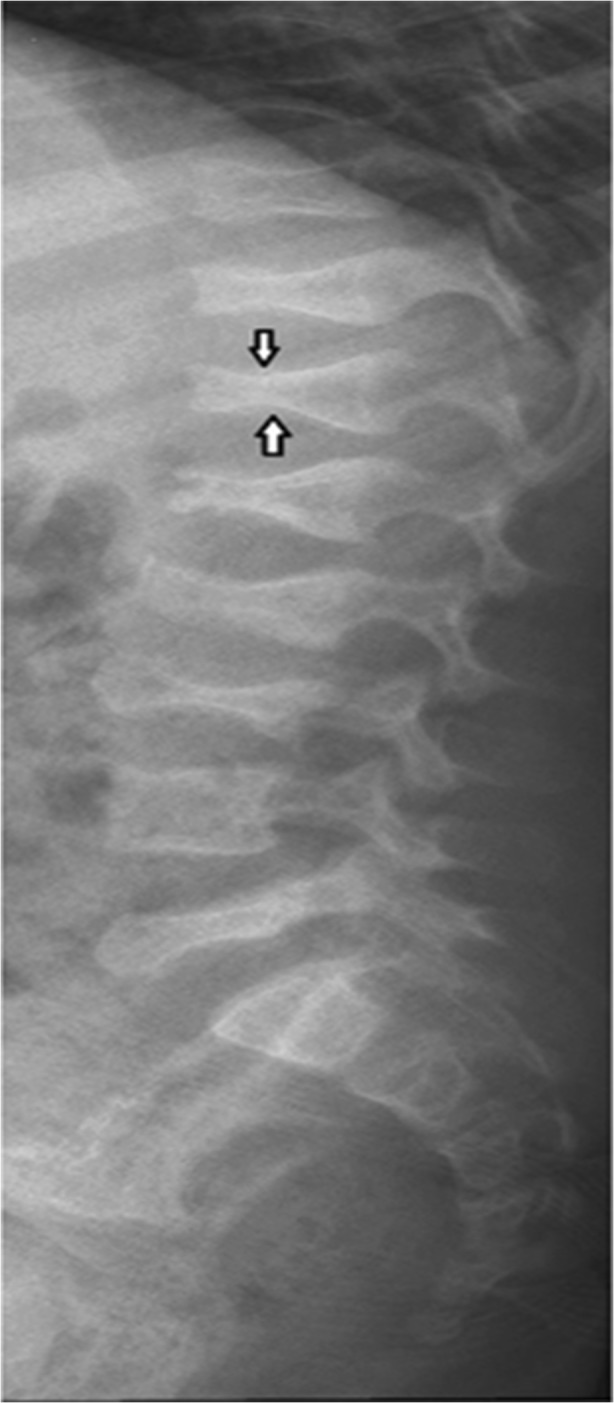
Lumbar and thoracic spine radiograph of a 6-year-old child with OI demonstrating anterior wedge compression fractures at multiple vertebrae [17]
